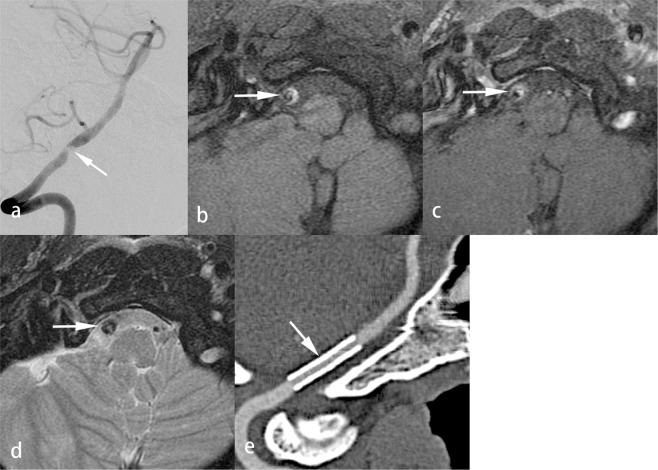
Soft lesion in a patient with dissection. A 44-year-old man suffered from recurrent headache and dysarthria for 1 week. Brain MRI showed multiple recent infarcts in the bilateral cerebellar hemispheres on DWI. Right vertebral angiogram showed acute dissection with 85% stenosis of the right distal vertebral artery (a). VW-MRI of the right distal vertebral artery showed a high-signal intramural hematoma on fat-suppressed T1WI (b, arrow) and T2WI (c, arrow) and focal strong enhancement of the intramural hematoma on contrast-enhanced fat-suppressed T1WI (d, arrow). During the PTAS, the PP was 3 atm. A 3.5 × 20 mm balloon-expandable stent was placed in the right distal vertebral artery. In a 4-year follow-up, CTA with curved multi-planar reconstruction showed good patency of the stent (e, arrow).
